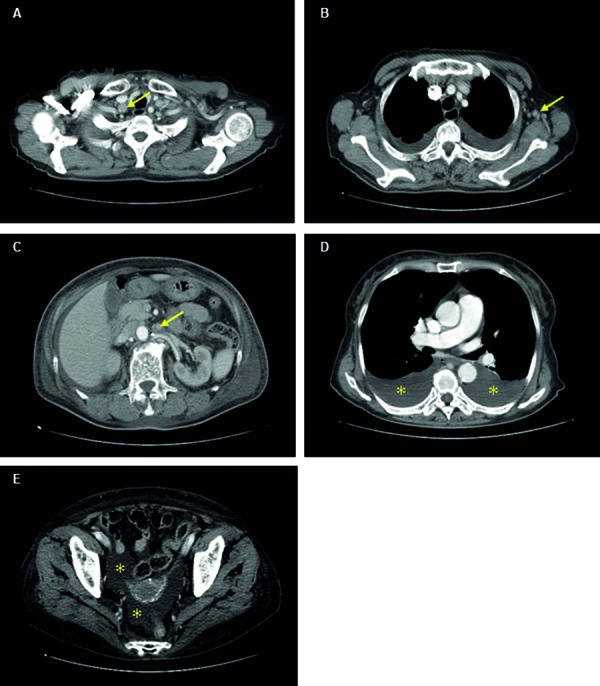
Radiographic findings. Whole body computed tomography showed multiple small lymphadenopathies (arrows) in the mediastinum (A), axilla (B), and para-aorta (C), bilateral pleural effusion (asterisks in D), and ascites (asterisks in E).
